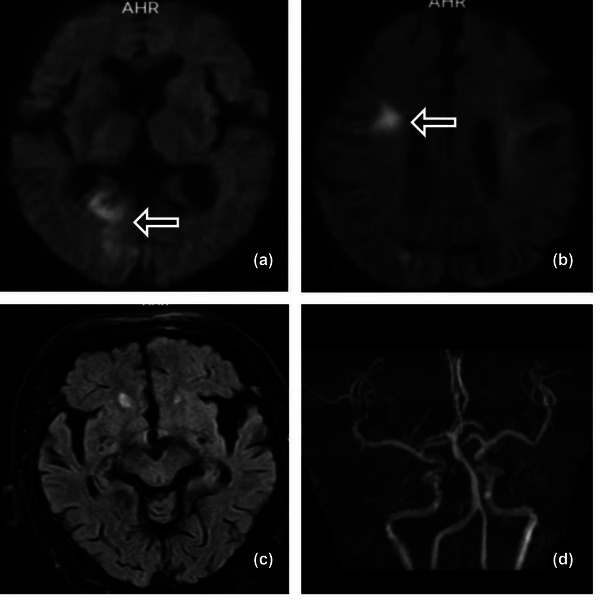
NVAF patient. A 78‐year‐old male was admitted 3.5 h after stroke and he was a NVAF patient. A+B: DWI, C: FLAIR, D: MRA. Multiple infarct in the right cerebral hemisphere. No obvious atherosclerotic stenosis in MRA.
